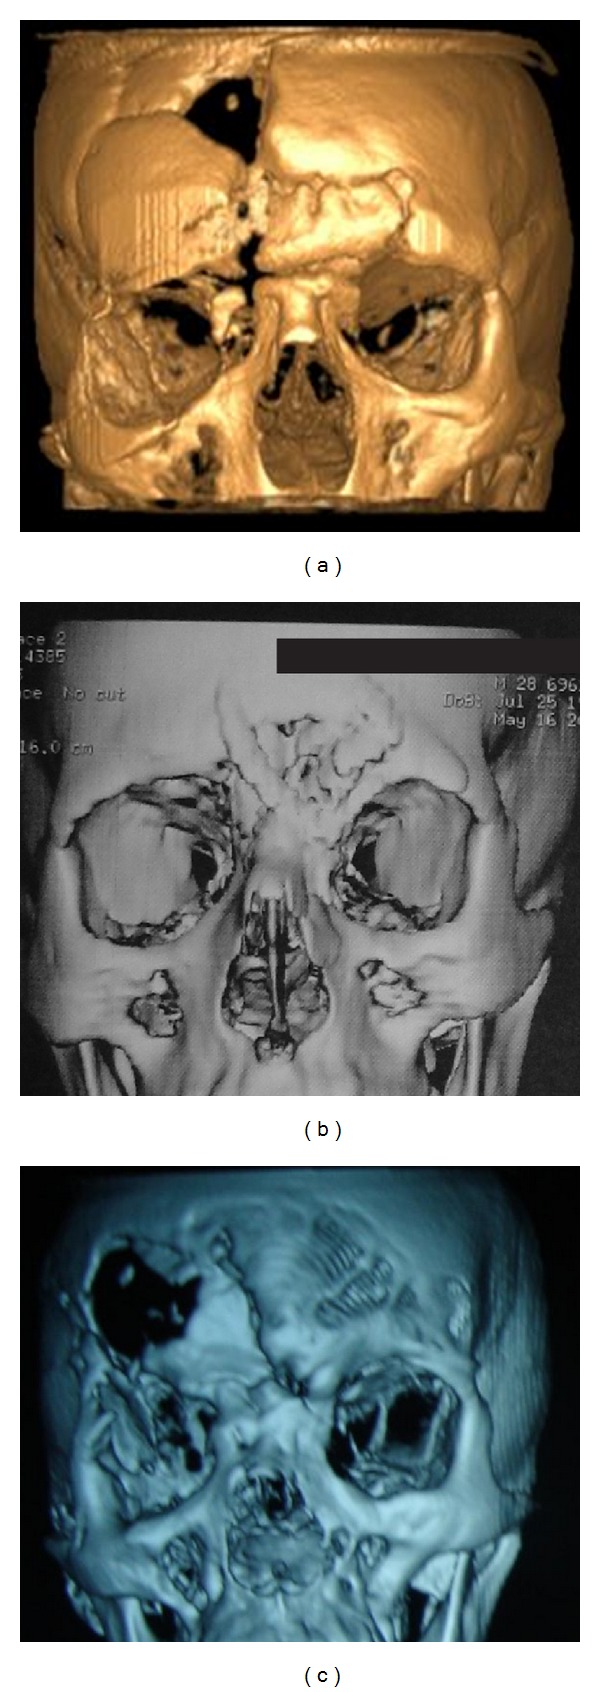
Preoperative 3D CT scans for Cases 1, 2, and 3 ((a), (b), and (c), resp.).
